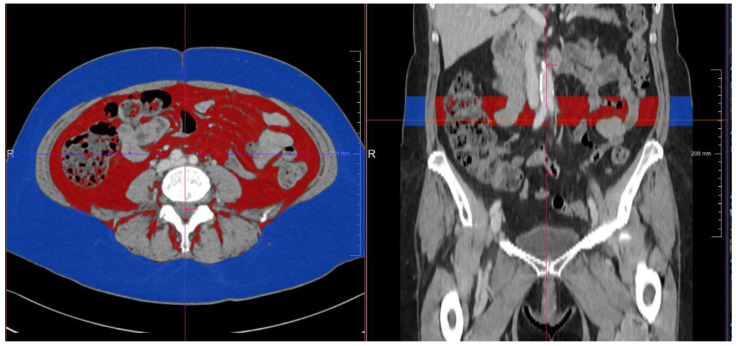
Depiction of computer-tomography (CT)-derived densiometric quantifications of subcutaneous (blue) and visceral (red) fat area performed on image slices between vertebral body L3 and L4. Quantifications were given as volume (mL) and included into statistical analysis in direct comparison to the BMI.
